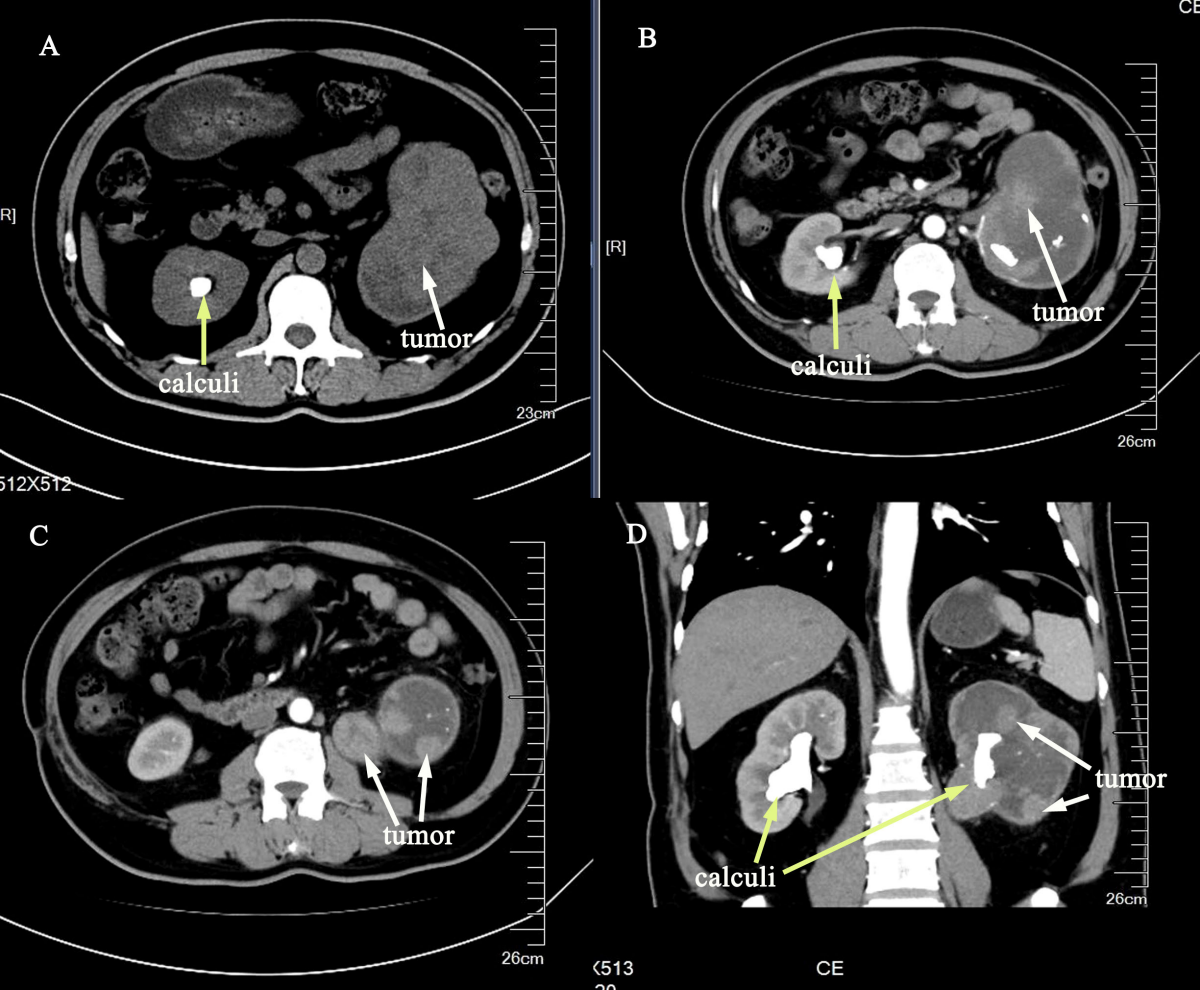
Contrast-enhanced CT scan with an irregular soft tissue mass shadow in the left renal pelvis, calyces and upper ureter (A–D), the mass was significantly enhanced in the arterial stage (B–D). Multiple nodular dense shadows were observed in both kidneys (A–D). (The tumor was showed by white arrows, and the calculi was showed by yellow arrows.).
